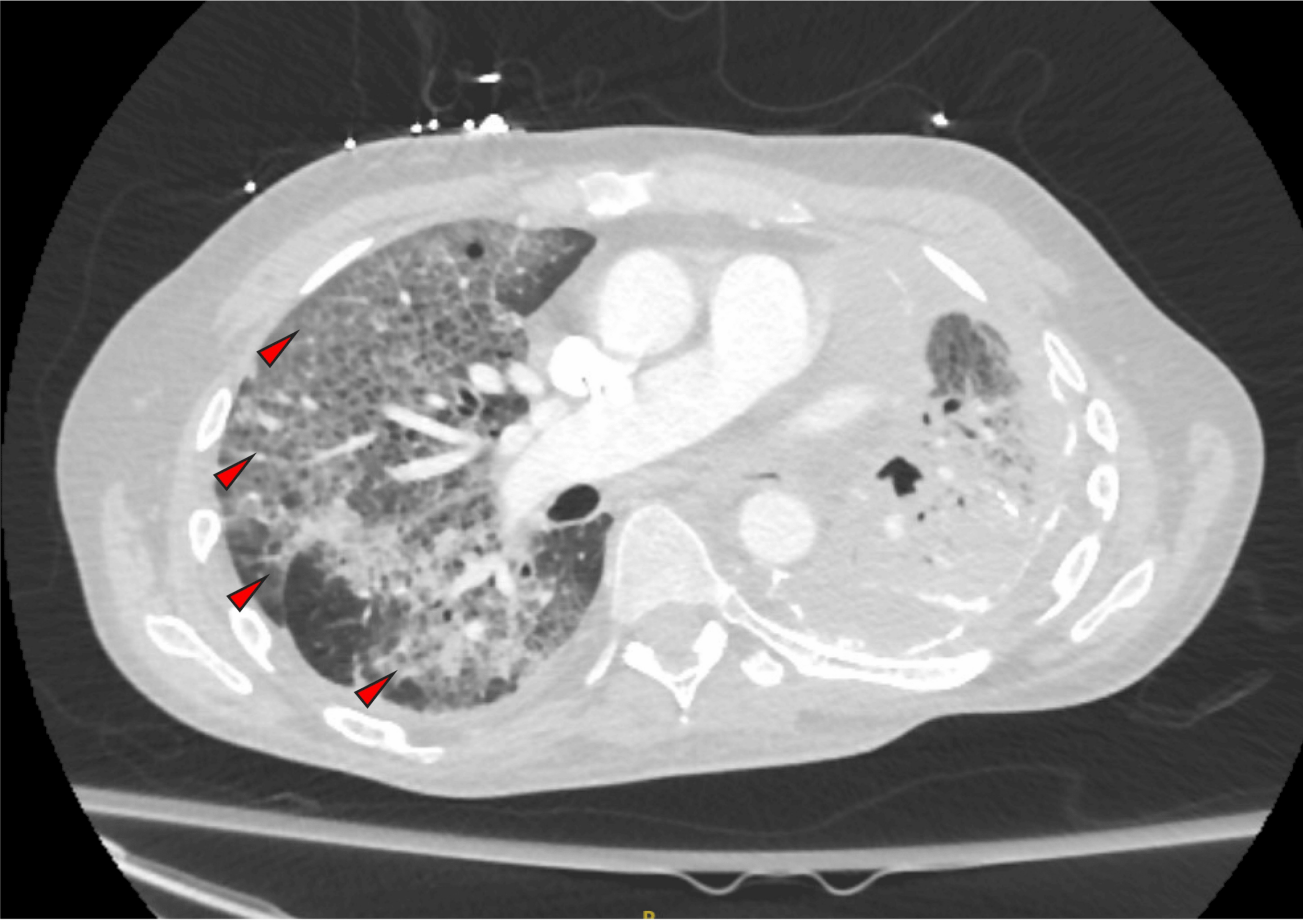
CT chest showed diffuse reticular and ground glass opacities. Left chest consolidations and pleural scarring are stable from previous study.
